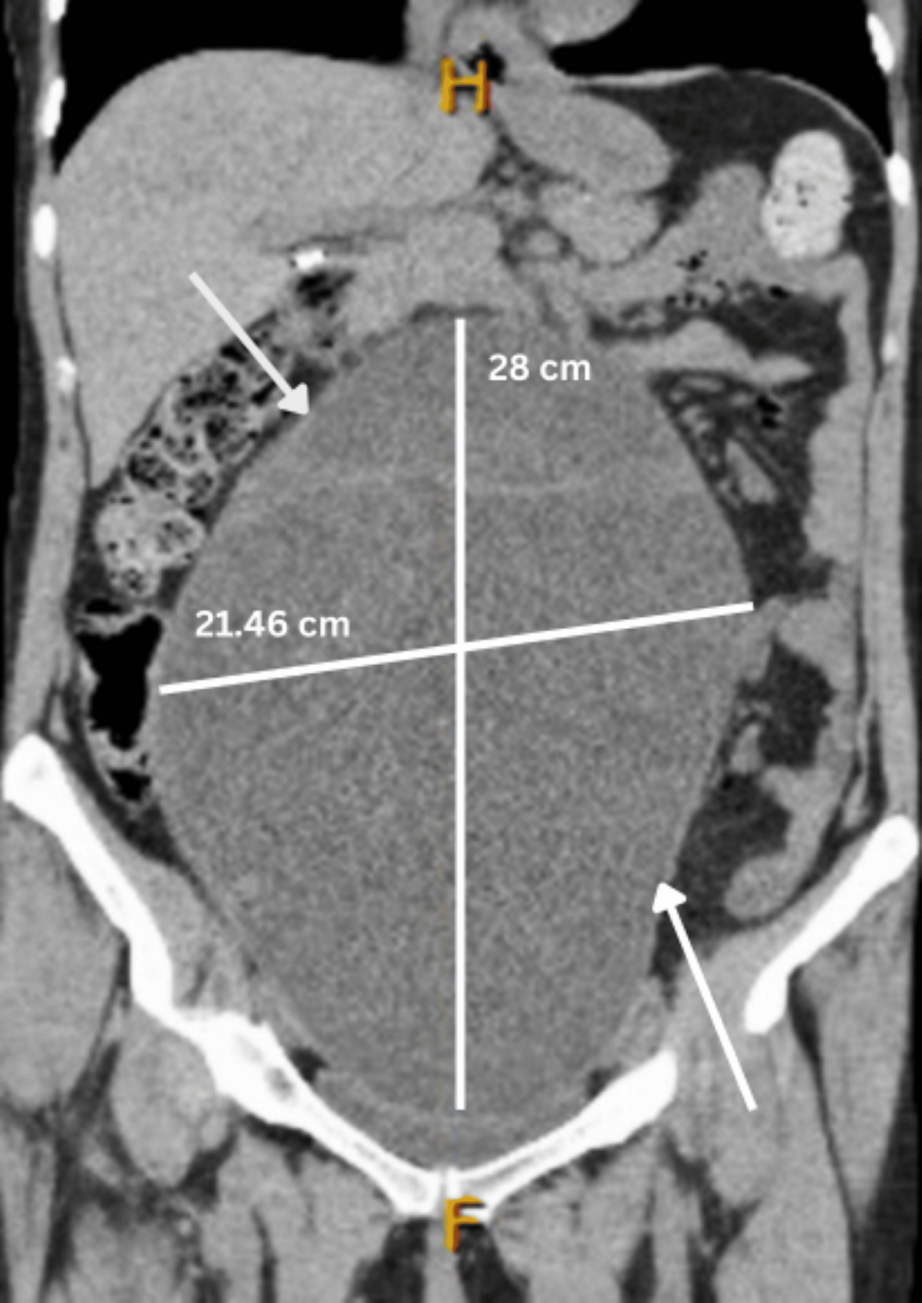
Coronal MRI image of the right borderline ovarian tumor measuring 21.46 cm x 28 cm.
